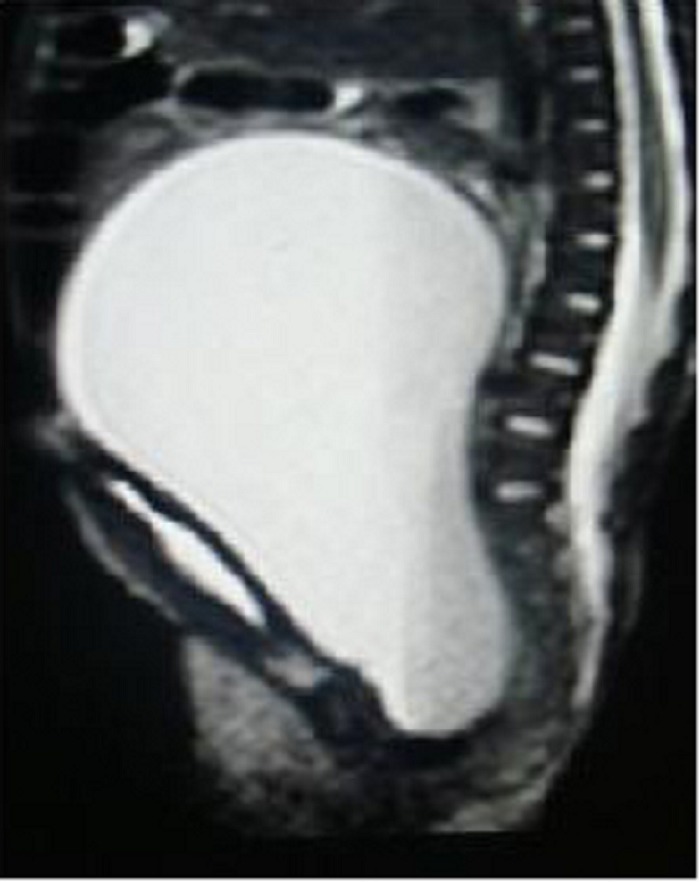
Figure 4: MRI of hydrometrocolpos with vaginal atresia.
